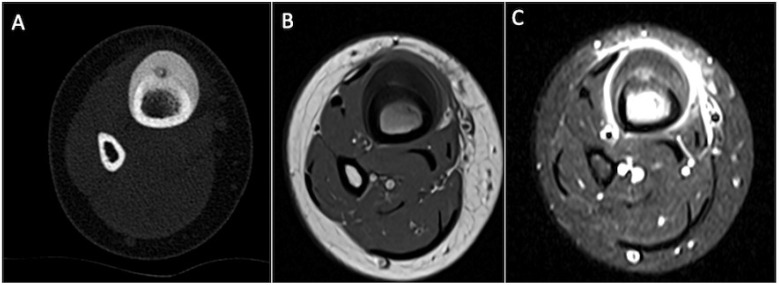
Lower leg axial CT image (A), axial T1-weighted (B), and STIR (C) axial MRI demonstrate the cortical thickening and extensive adjacent periosteal and soft tissue oedema.
